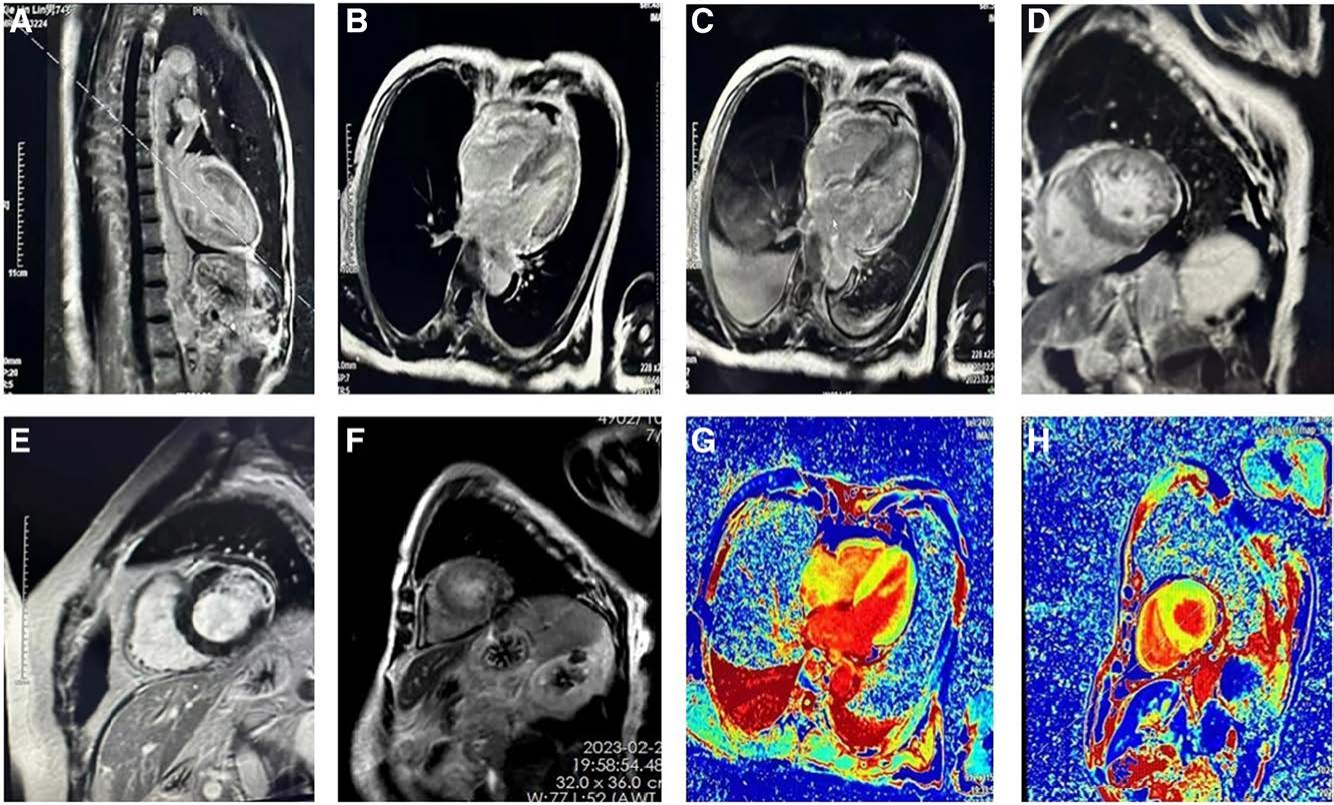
(A–H) Changes of cardiac magnetic resonance imaging at admission.
